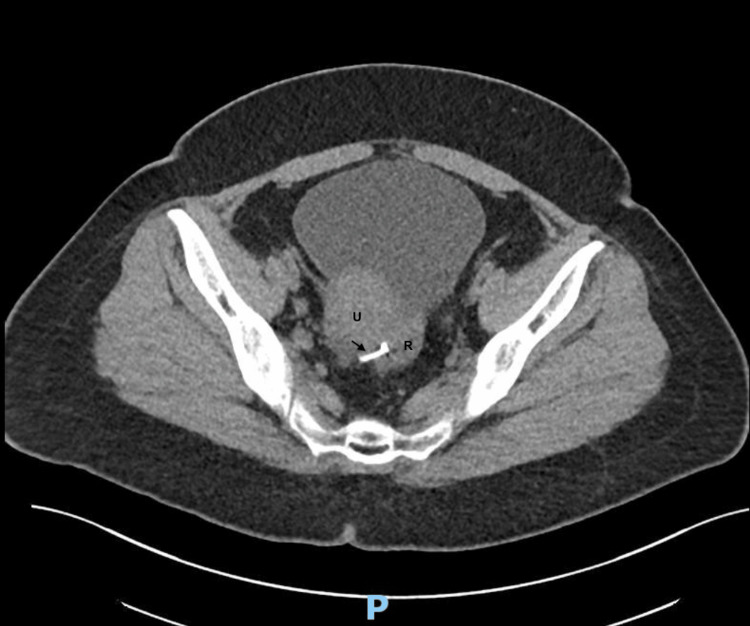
A CT scan of the KUB (transverse view) shows the intrauterine device (arrowed) has migrated and is now located between the body of the uterus (labelled U) and the adjacent rectosigmoid colon (labelled R). KUB: kidney, ureter, and bladder
